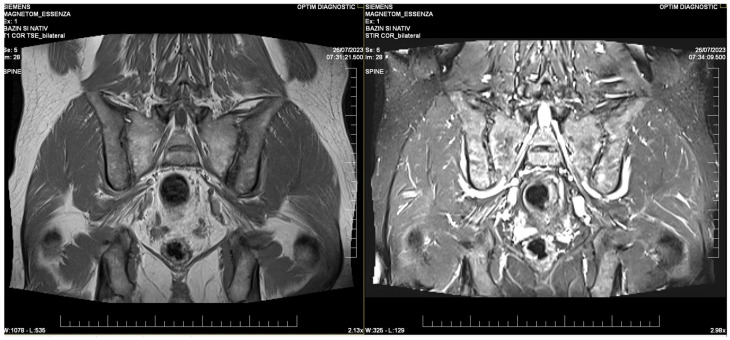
MRI imaging of the sacroiliac joints demonstrates bilateral sacroiliitis. The T1-weighted image (left) reveals subchondral sclerosis, joint space narrowing, and erosions, all indicative of chronic structural changes. The STIR sequence (right) highlights active inflammation, showcasing bone marrow edema visible as areas of hyperintense signal.
